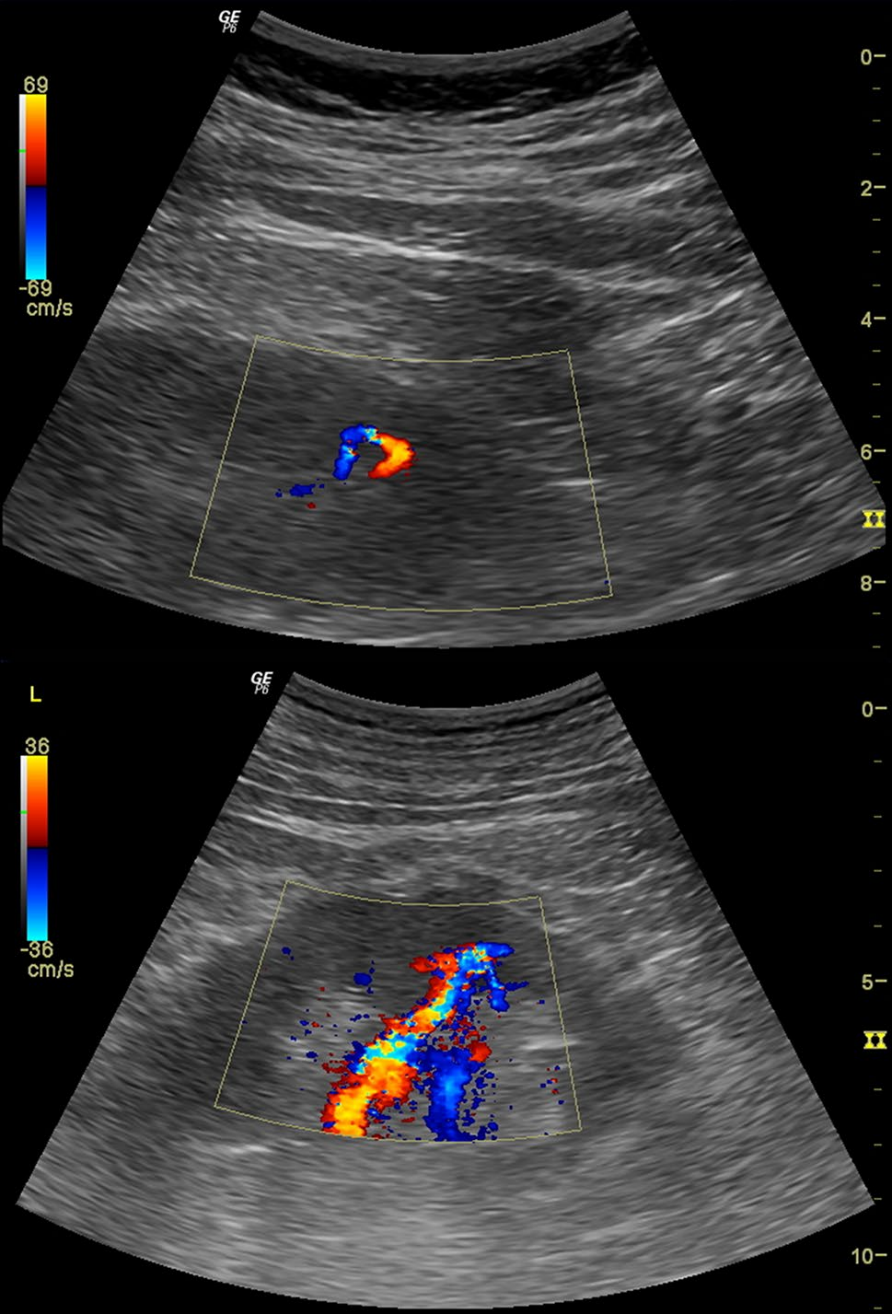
Renal biopsy-related arteriovenous fistulas detected in color Doppler
